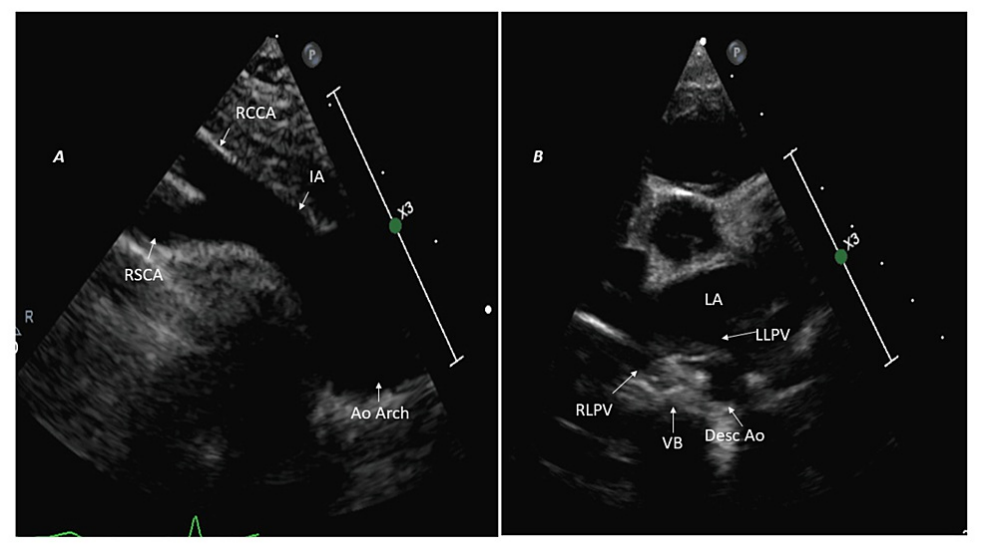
Left aortic arch with normal branching pattern and left-sided descending aorta (A) Suprasternal short-axis view: first branch (IA) courses toward the right and bifurcates. (B) High parasternal short-axis image: left-sided descending aorta (Desc Ao) located posterior to left lower pulmonary vein (LLPV) on the left side of the vertebral body (VB). Ao Arch: aortic arch; IA: innominate artery; RSCA: right subclavian artery; RCCA: right common carotid artery; RLPV: right lower pulmonary vein; LA: left atrium.
